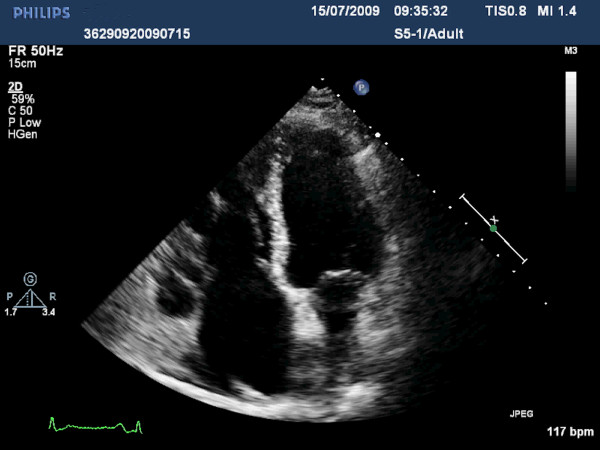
Apical four-chamber view in systole displaying an opened and retracted tricuspid valve, while mitral valve is closed.
